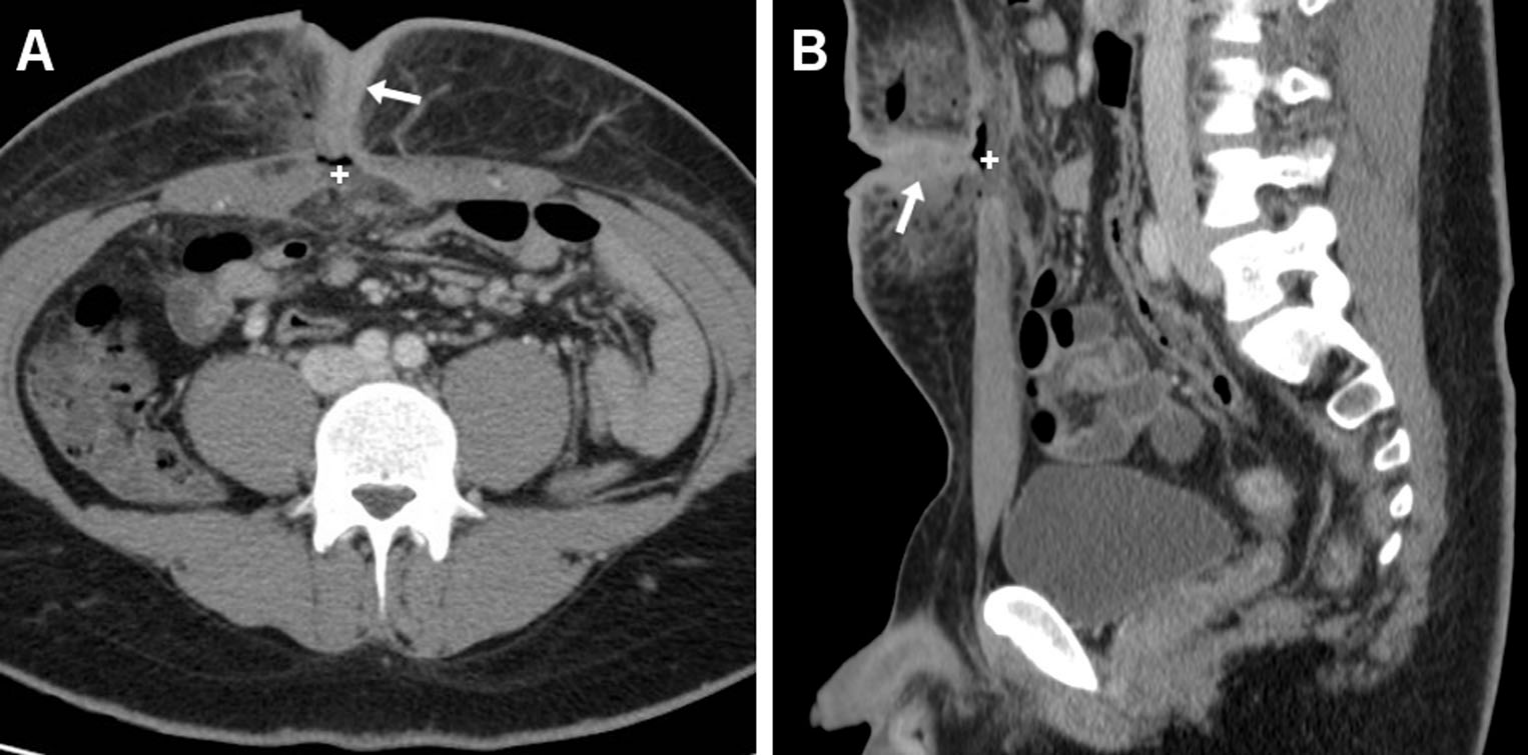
Another case of fistulising post surgical infection in a 41-year-old male after VHR of recurrent incisional hernia. Pus-yielding wound infection corresponded on axial (a) and sagittal (b) post-contrast CT images to a small-sized collection with mixed air (+) and fluid (*) content in the typical midline site, abutting the PM ventrally. A characteristic tram-track fistula (thick arrows) drained the collection towards the skin, and was a surrounded by extensive inflammatory stranding of the subcutaneous fat
